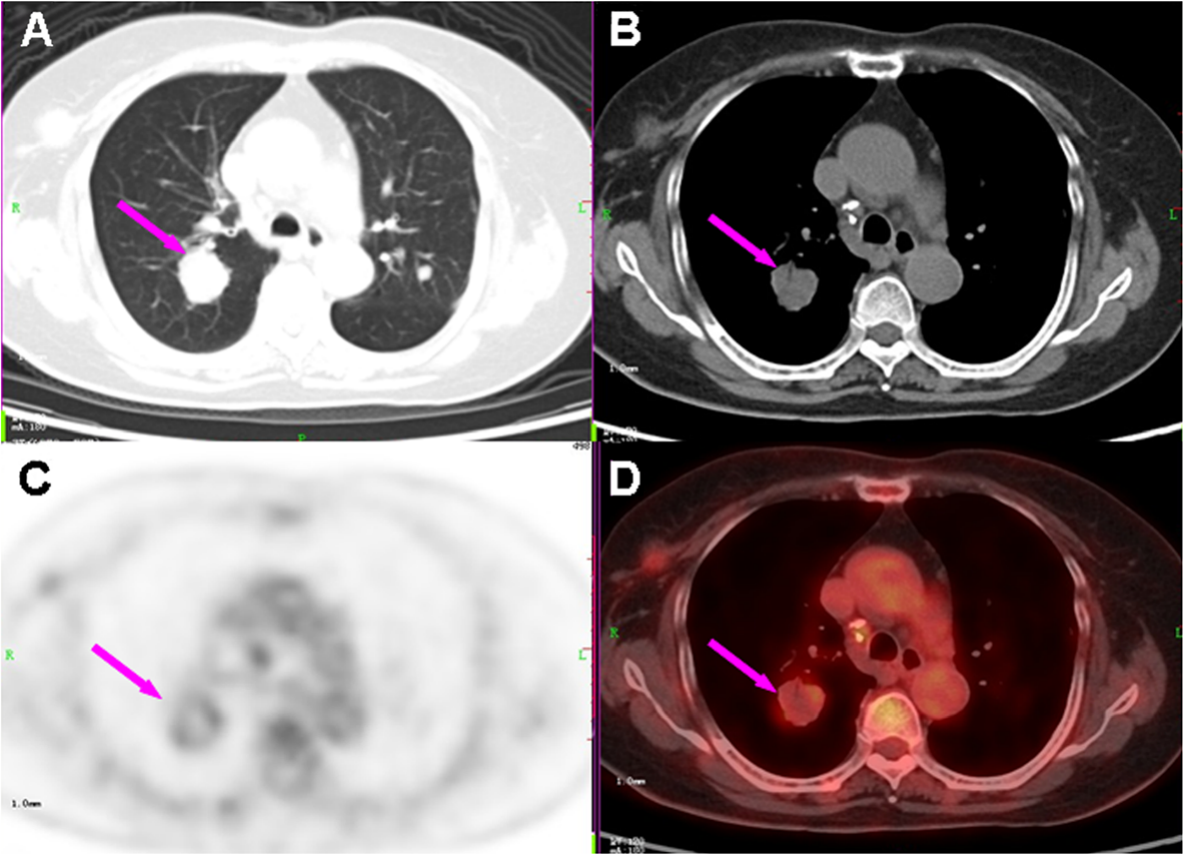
Computed tomography (CT) lung window (A), CT mediastinal window (B),^18^F-fluoro-deoxyglucose positron emission tomography (FDG-PET) (C), and fused PET/CT (D) images of the pulmonary lesion. Axial images show a round, relatively isodense lesion with mild ring-like FDG uptake (SUVmax of 2.96) in the right upper lobe of the lung (arrows).
